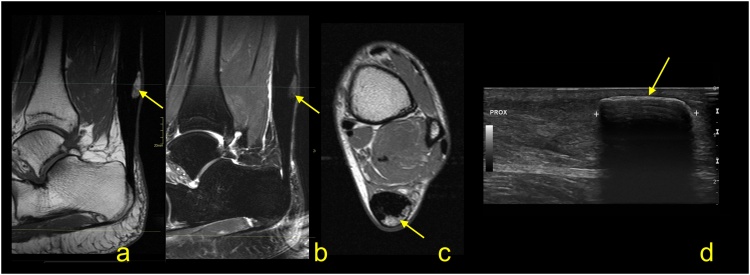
Achilles tendinopathy after many years. More pain after walking in the last 2 months. The MRI (a and b) and ultrasound (c) revealed the presence of superficial calcification (arrow) within a thickened Achilles tendon. A – PD-weighted, b- T2-weighted with fat suppression, c- PD-weighted, d- ultrasound, longitudinal scan of the Achilles tendon.
